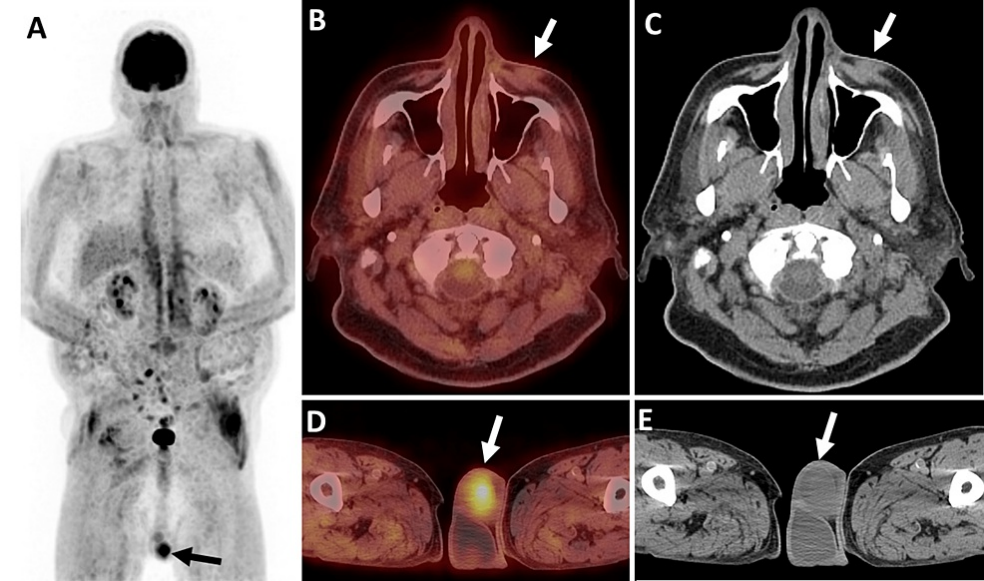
Re-staging 18F-FDG PET/CT: (A) maximum intensity projection, (B and D) trans-axial fused PET/CT, and (C and E) unenhanced axial CT images demonstrating significant interval decrease in size and FDG uptake of the left premaxillary soft tissue (SUVmax 1.5, white arrow in B and C) without hypermetabolic lymph nodes in the neck, chest, and abdomen. Incidentally seen is an asymmetrical intense FDG uptake in the left testicle (SUVmax 10.3, white arrow in D and E). 18F-FDG PET/CT: 18F-fluorodeoxyglucose positron emission tomography/computed tomography
